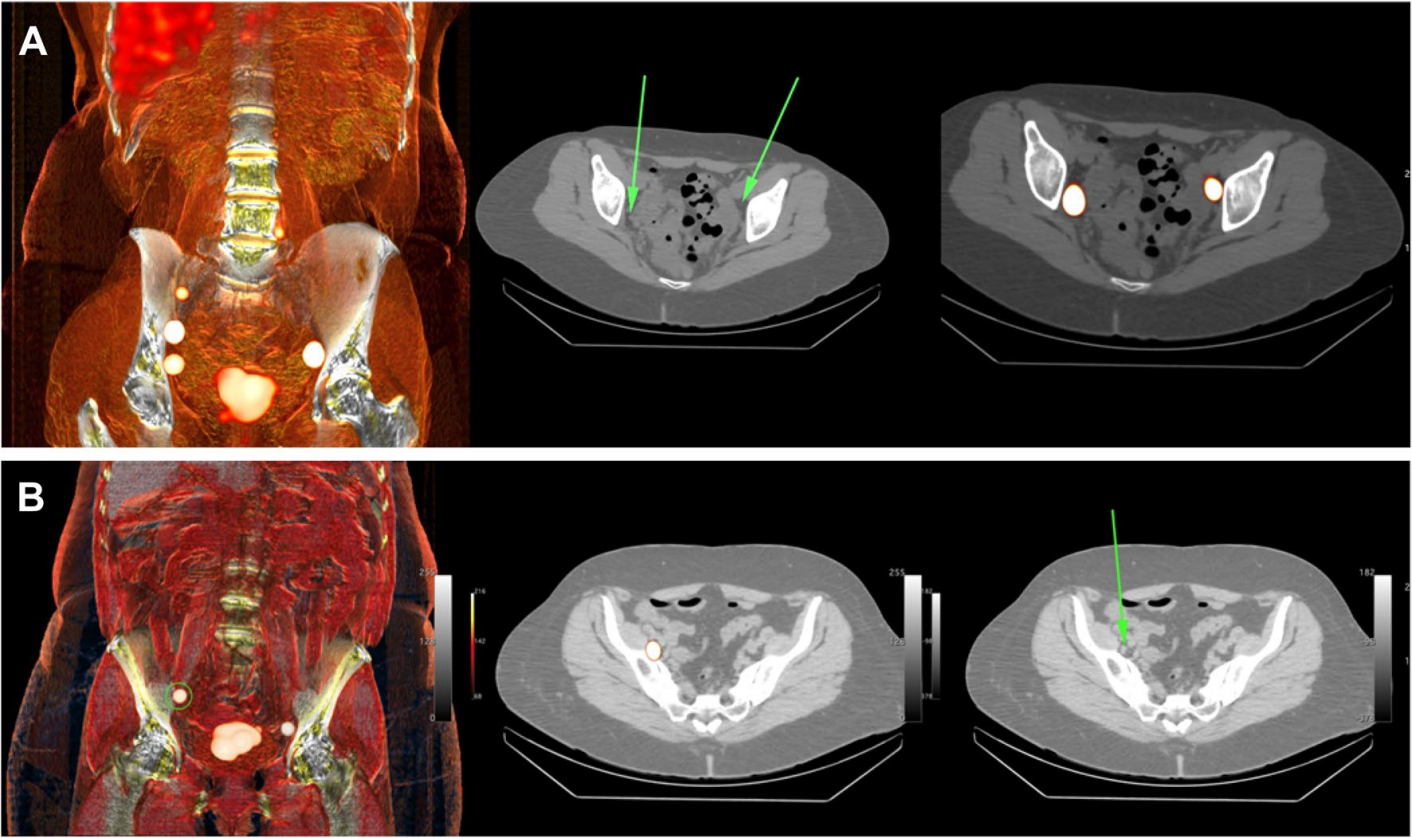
Preoperative sentinel lymph node mapping using nuclear imaging. A SPECT/CT imaging in cervix cancer, showing the sentinel node locations on a surface render overview (left) and detailed axial slices (middle and right). B SPECT/CT imaging in endometrial cancer, showing the sentinel node locations on a surface render overview (left) and detailed axial slices (middle and right)
